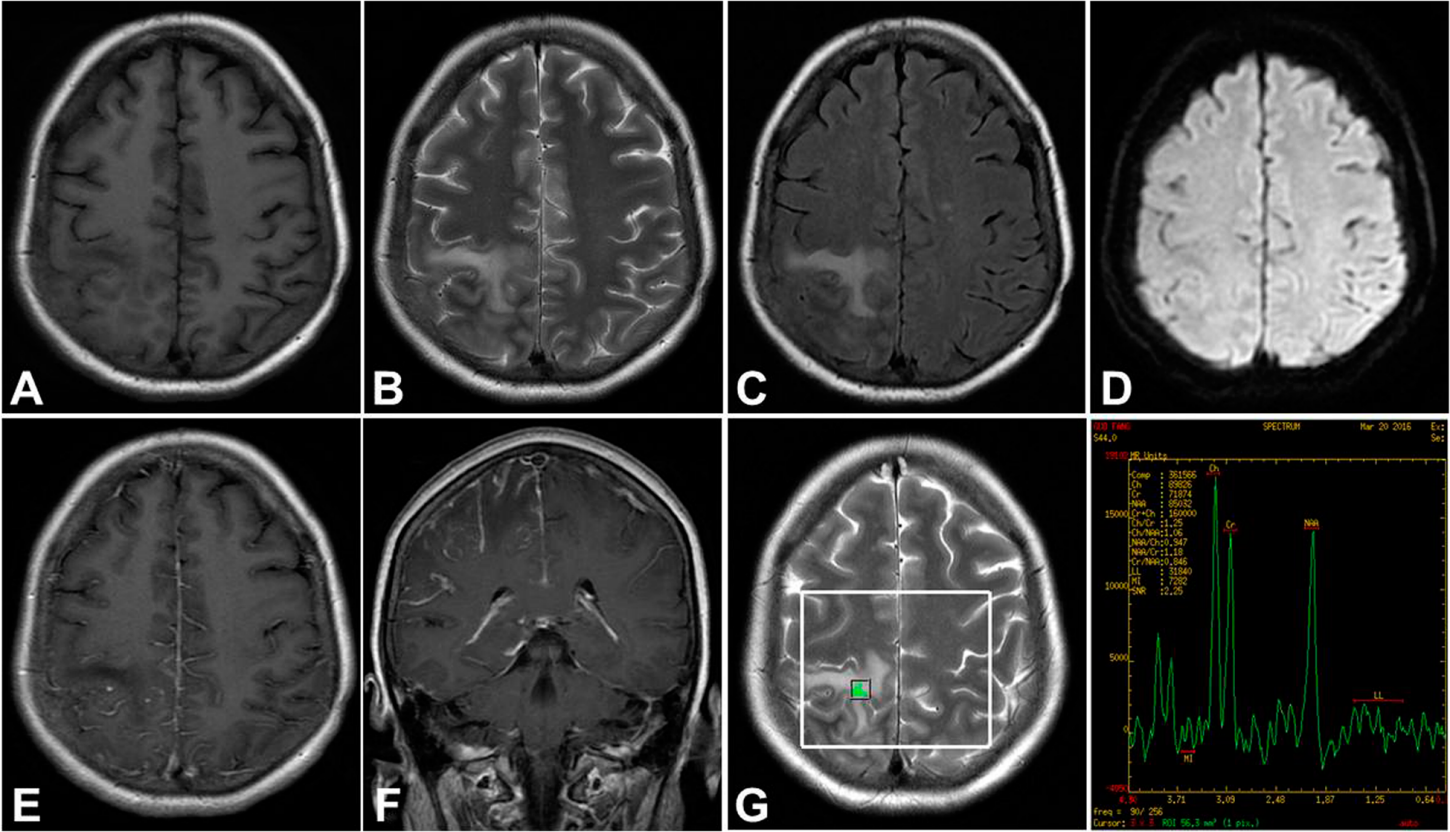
MRI on the 1st hospital day showed a lesion on *right* parietal lobe that was hypointensity on T1-weighted (a), hyperintensity on T2-weighted images (b), high signal on FLAIR (c), and normal signal on DWI (d). MRI enhancement showed contrast enhancement along the surface of swollen gyrus lesion (e) and (f). MRS showed slightly decreased NAA and increased Cr and Cho peak on the lesion (g)
